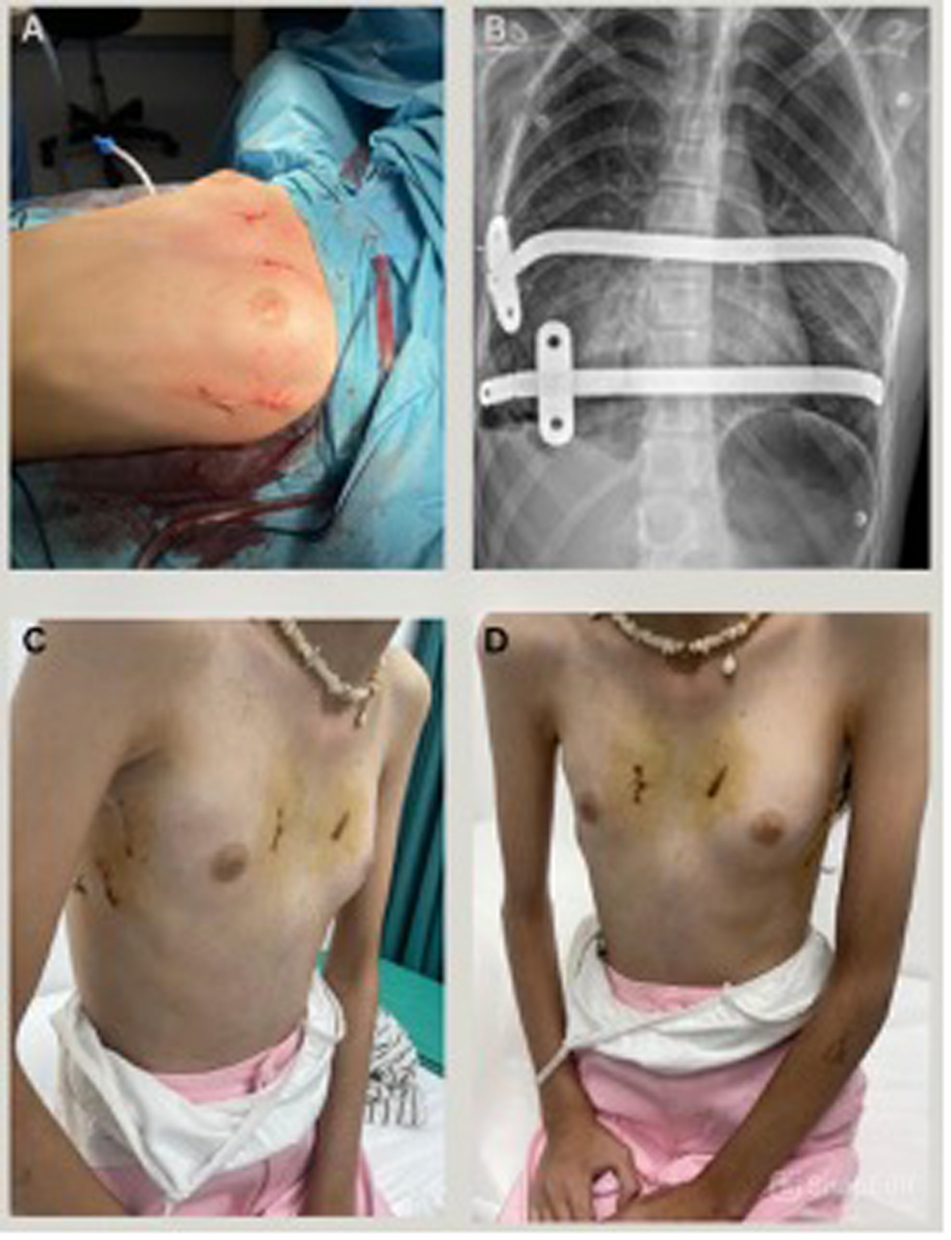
(A) Supplementary. Post operative | case 2. (B) Supplementary. Post operative x-ray | case 2. (C) Supplementary. 30 days postoperative lateral view | case 2. (D) Supplementary. 30 days postoperative anterior view | case 2.
